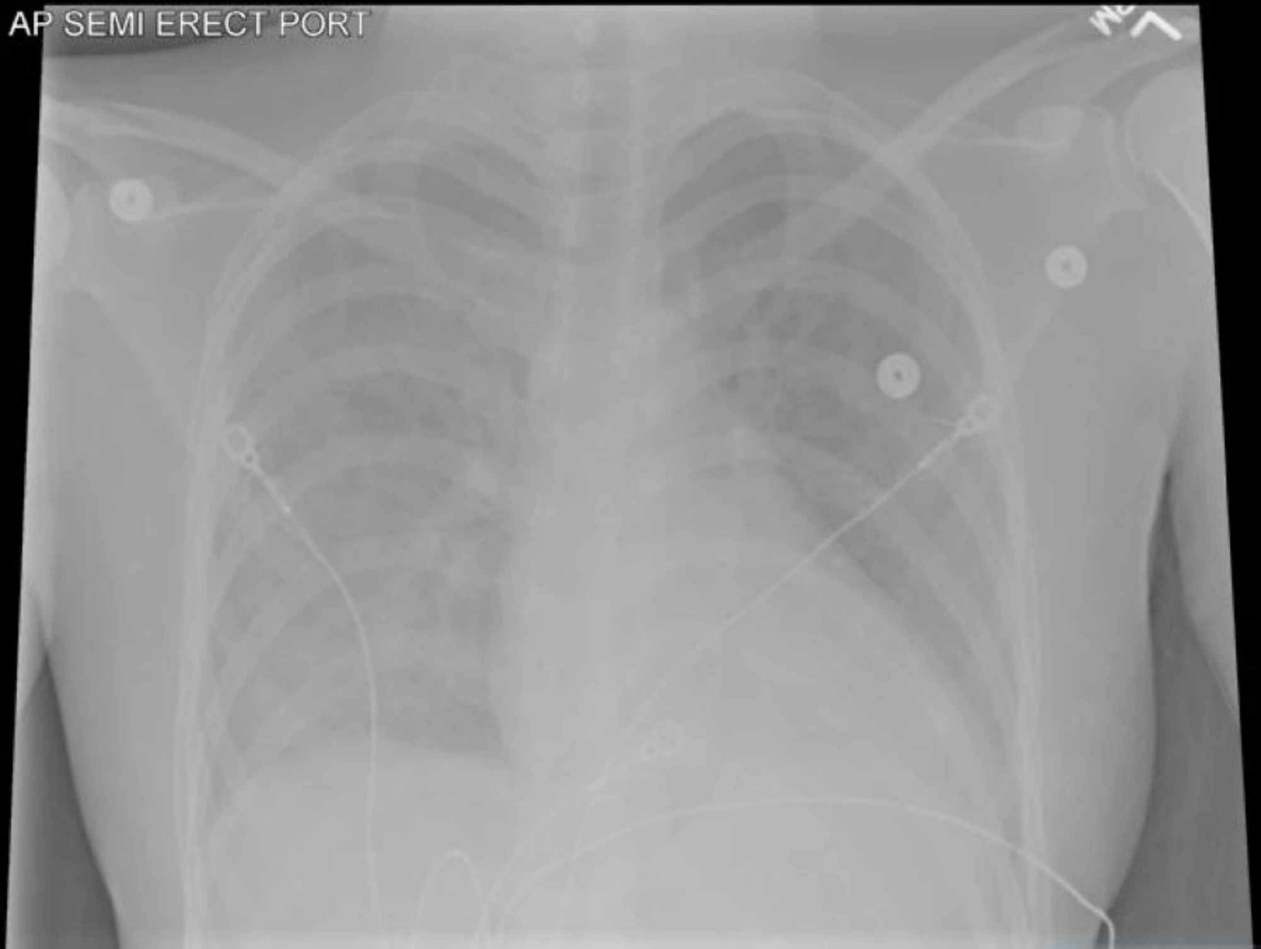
Portable antero-posterior chest radiograph showing diffuse ground-glass opacity in the right lung
